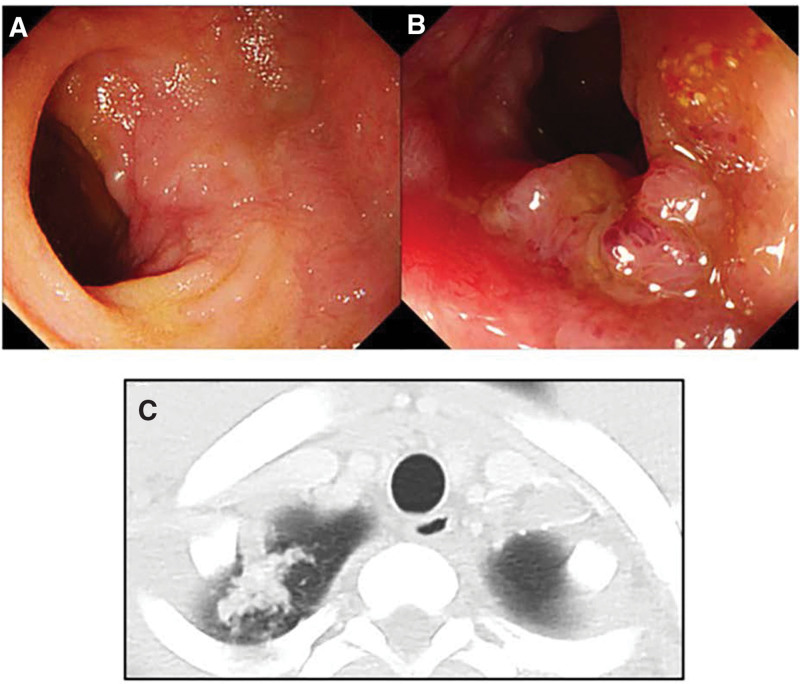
(A and B) Colonoscopy findings: improved lesions and a remained active ulcer in the terminal ileum. (C) Chest computed tomography findings: a worsening nodular lesion 17 wk after the anti-TNF-alpha agent initiation.
